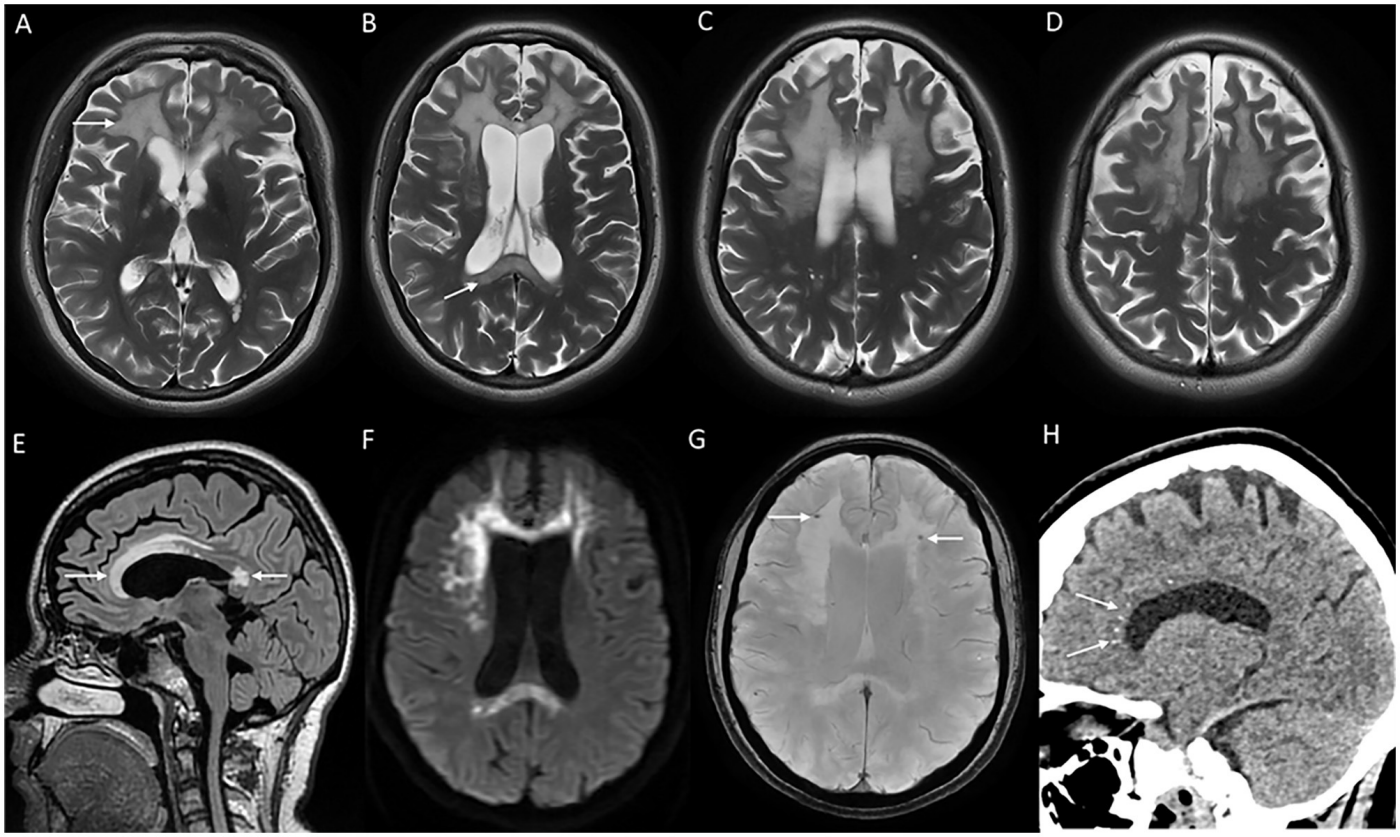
MRI brain findings of a patient with *CSF1R*-related leukoencephalopathy with T2W axial images (A–D) reveal confluent near symmetric bifrontal periventricular and deep white matter signal changes including the splenium of the corpus callosum [arrow in (B)], T2 FLAIR sagittal images (E) with signal changes involving rostrum, genu, anterior part of the body, and splenium of the corpus callosum; diffusion-weighted images (F) with heterogeneous high signal in frontal white matter and splenium; SWI (G) with microcalcifications in the frontal subcortical white matter, and CT coronal image (H) demonstrating stepping stone pattern of calcification involving the anterior part of the corpus callosum.
